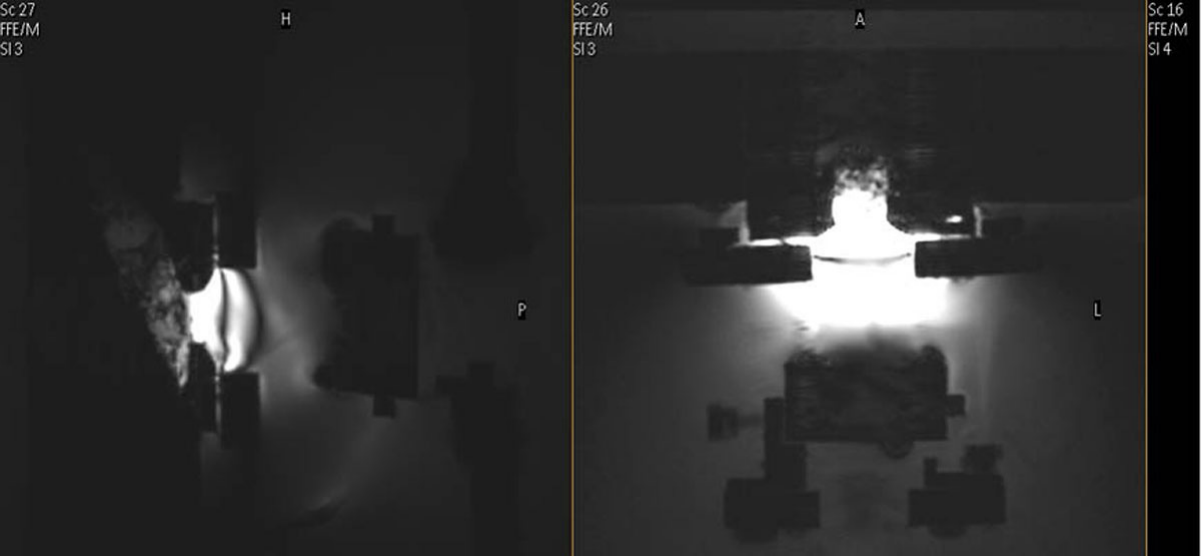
MR images showing the transducer used during trials
